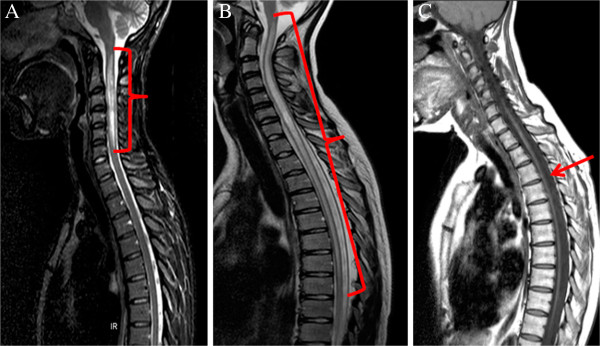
Longitudinally extensive transverse myelitis (LETM) and atrophy of spinal cord following LETM. Spinal cord MRI: sagittal T2WI (A and B) and T1WI (C) from three anti-AQP4- antibody positive NMO patients. A. MRI showing cervical spinal cord LETM with swelling. B. MRI showing LETM of cervical and upper 2/3 thoracic spinal cord. C. Severe atrophy of spinal cord as a consequence of recurrent LETM after 6 years duration of disease.
